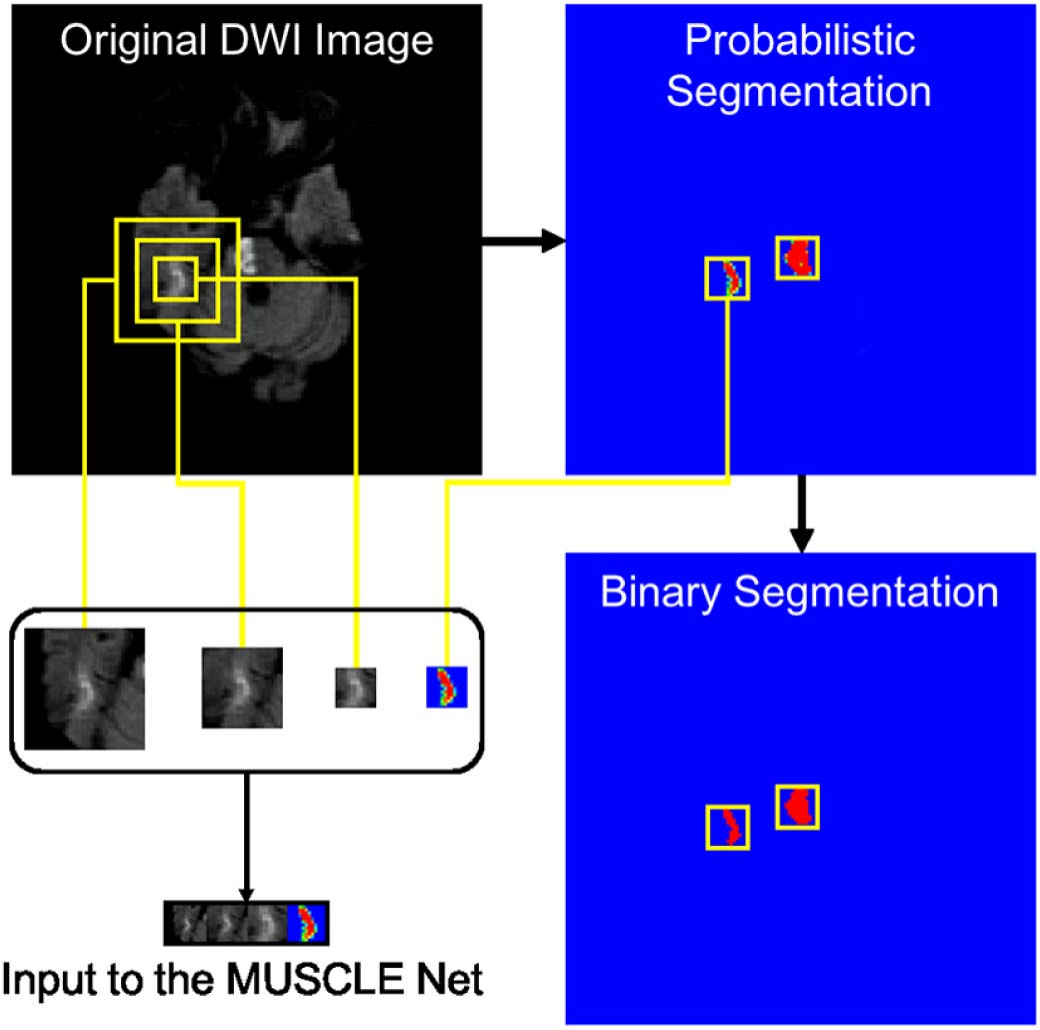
The derivation of the input to the MUSCLE Net. The probabilistic segmentation is obtained from the EDD Net. The binary segmentation is obtained by thresholding the probabilistic segmentation. Candidate small blobs are detected in the binary segmentation. The corresponding patches are extracted in the original DWI in multiple scales and the probabilistic segmentation map. They are then resized and concatenated resulting in the input to the MUSCLE Net.
